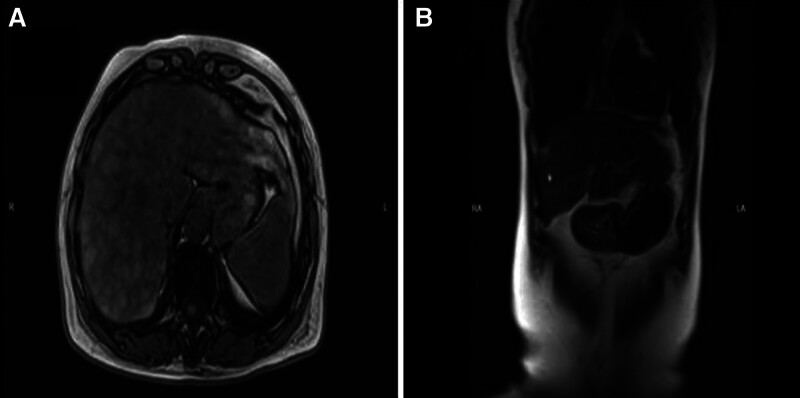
(a) Computed tomography image and (b) Magnetic resonance image show that liver profile was contoured like a wave. Imbalance between left and right liver lobes, widened liver fissure. Nodular liver cirrhosis was thought.
